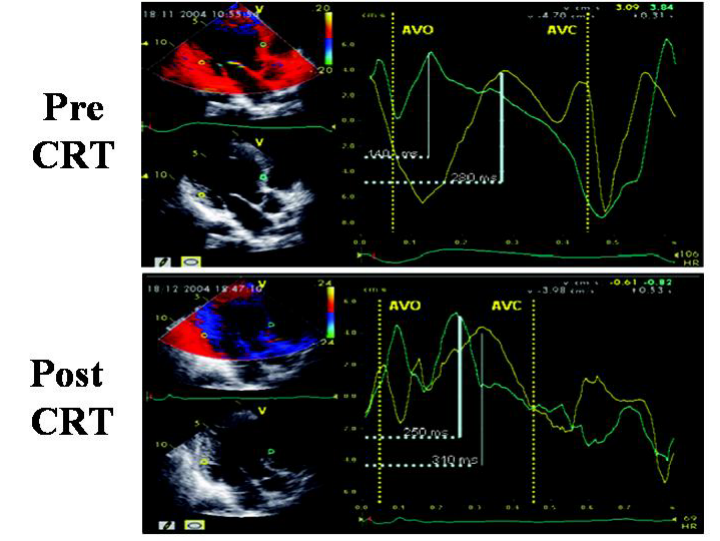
Sample of time to peak (Ts) measured by off-line color TVI pre and post CRT at the level of anterior septum and postero-lateral wall, in apical 5-chamber view. Aortic valve opening (AVO) and closure (AVC) are derived by previous placement of markers on the onset and the end of LV Doppler outflow. Pre-CRT Ts of postero-lateral wall (upper panel) is clearly delayed in comparison to Ts of anterior septum. Post-CRT the time duration of Ts between the two walls is clearly shortened (lower panel). (Modified from Innelli P et al, *Echocardiography *2006;23:709–716)
